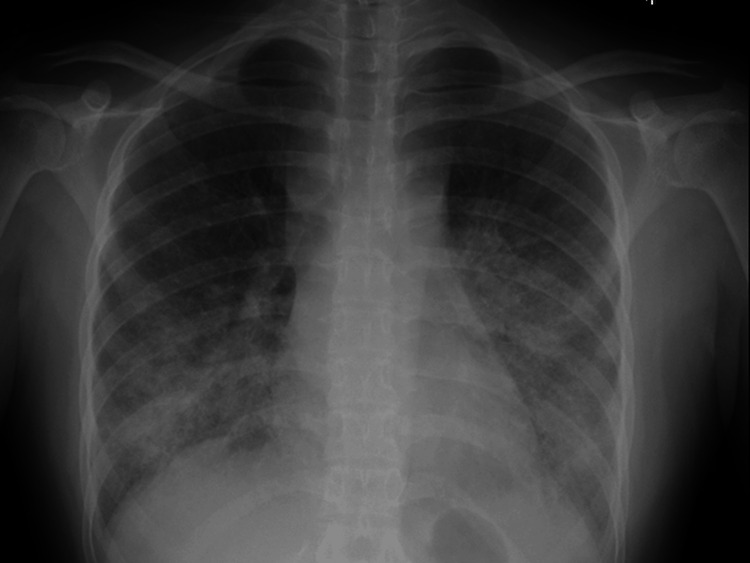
Chest radiograph Chest radiograph showing bilateral diffuse opacities with lower lung distributions.
